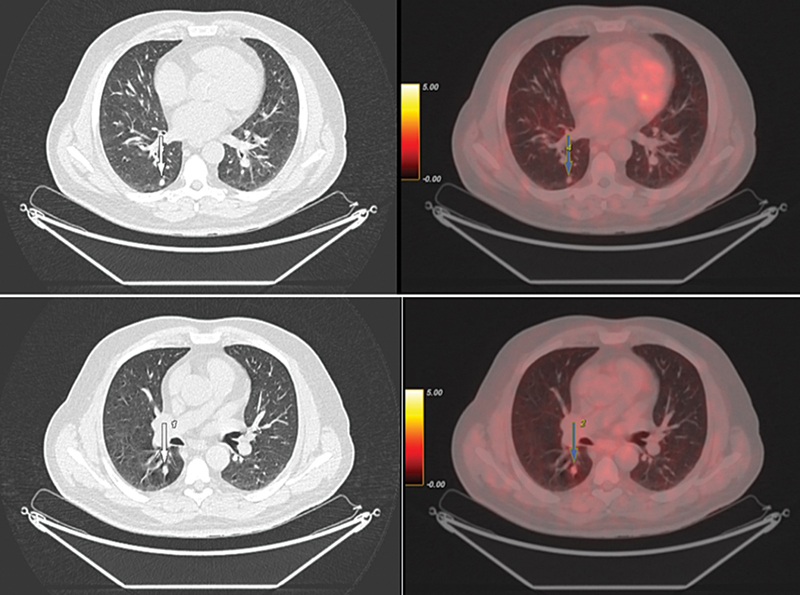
Transaxial CT and fused PET/CT images demonstrated lung nodules; some of them showed mild
